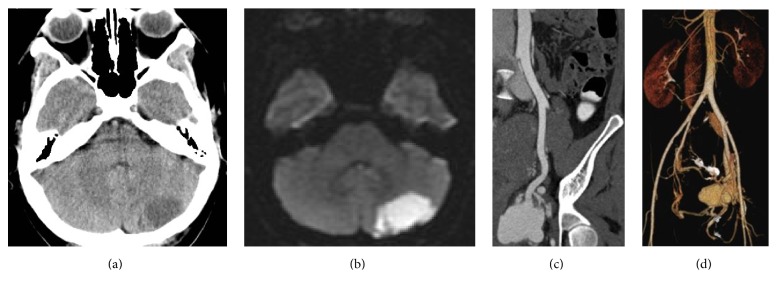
(a) Noninjected head CT with hypodense left hemicerebellar lobe. (b) Brain MRI: diffusion sequence confirming the hyperintense left hemicerebellar ischemic lesion. (c) Coronal oblique view of the pelvic mass in the arterial phase. (d) Volume rendering showing the mass with its arterial supply and venous drainage. Iliac vein thrombosis is not shown in this figure.
